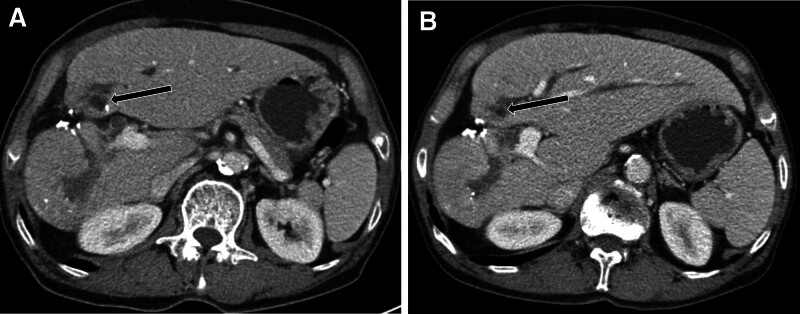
(A) A 1.5 cm–sized low-density lesion in S4b of the liver on 5-mo postoperative CT scan (arrow). (B) Gradual decrease in the size of the lesion over time (arrow). CT = computed tomography.
